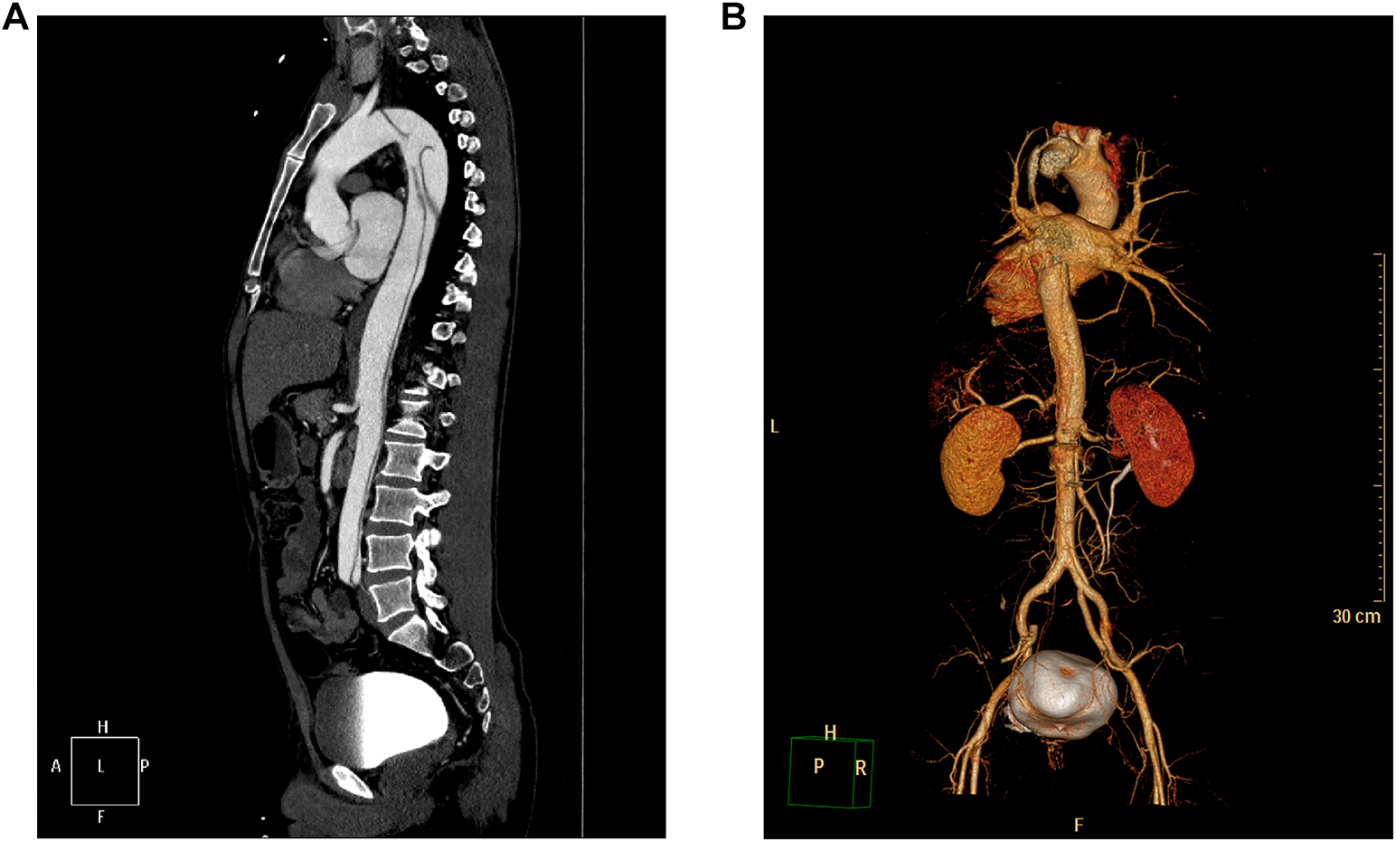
CTA showing medial migration of the intimal sheet from the descending thoracic aorta to the level of both common iliac arteries, and the lumen showing “double-lumen” changes, consistent with aortic dissecting aneurysms (Stanford type B). (A) Maximal intensity projection of the sagittal section of the aorta. (B) Three-dimensional reconstruction of the aorta.
